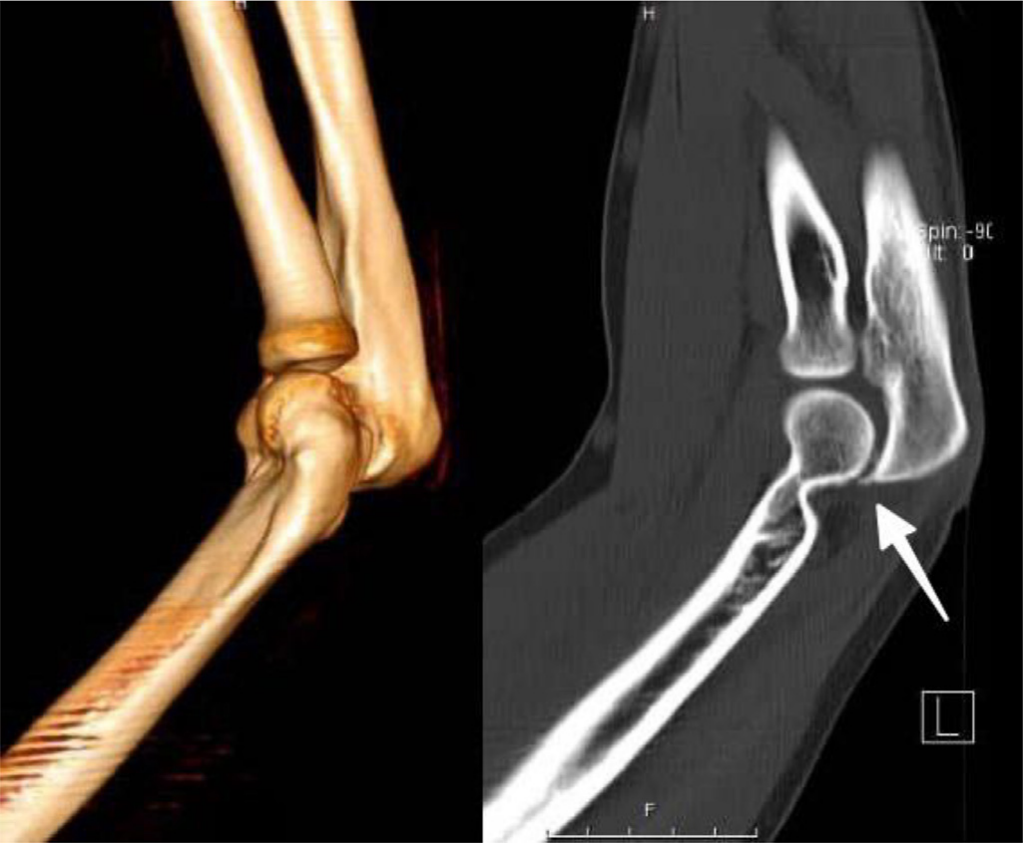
Preoperative CT imaging of the elbow joint. Left: 3D volume-rendered CT reconstruction in lateral view. Right: Sagittal CT reformation in bone window showing normal articulation between the olecranon and the trochlea (white arrow) without evidence of dislocation at initial presentation.
